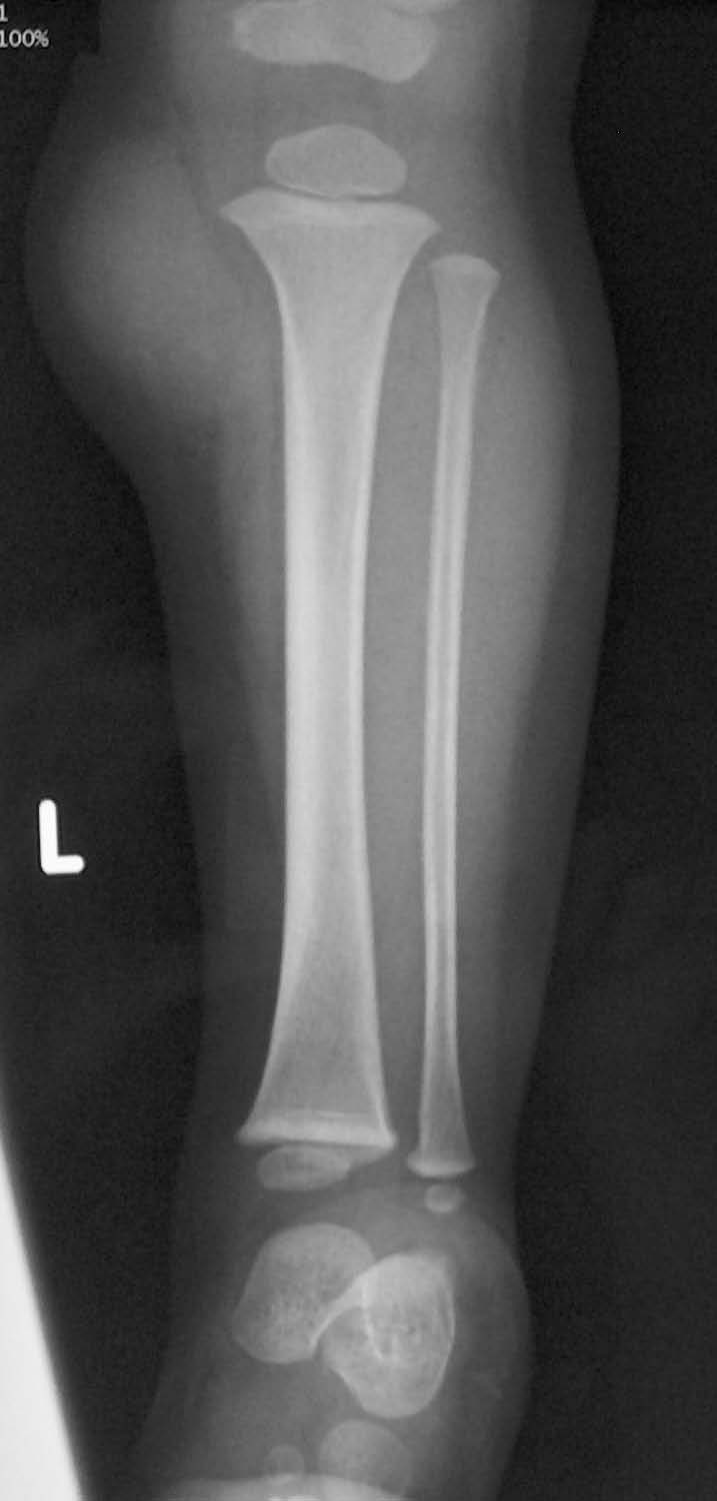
Case 1- Plain radiograph of the left tibia, demonstrating the mass in the inner-upper part.
